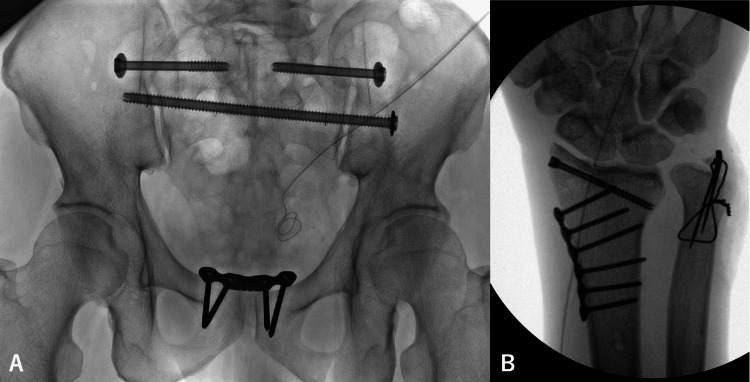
Postoperative X-ray (A) Plate and screw fixation of the pelvis. (B) Plate fixation and pinning of the right wrist joint.
